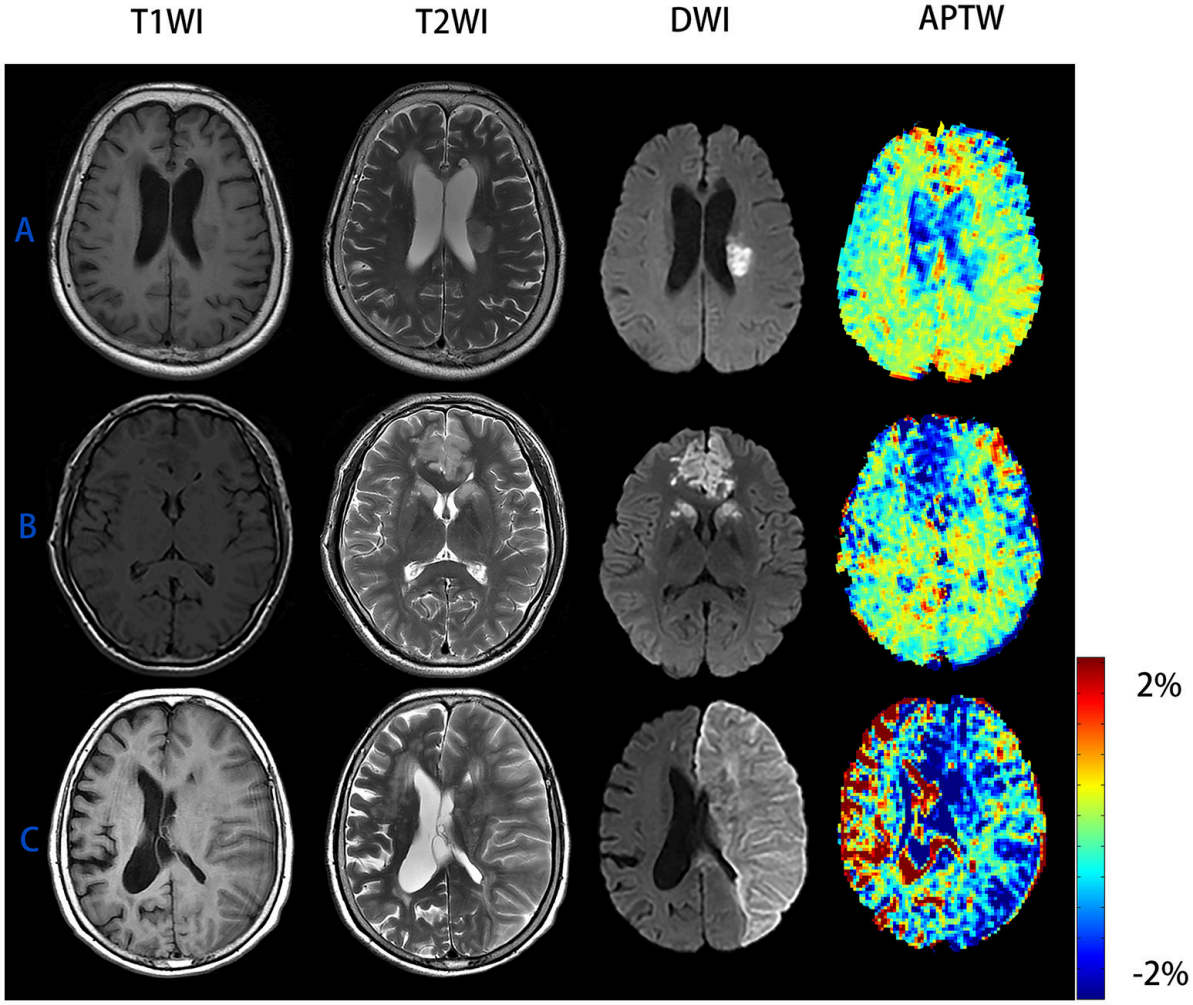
Conventional MR images and APTW images of patient with AIS of different NIHSS scores and mRS scores. (A) M/65 years, NIHSS score was 3 and 90-day mRS score was 0, ΔAPTW = −0.37%. (B) F/69 years, NIHSS score was 5 and 90-day mRS score was 2, ΔAPTW = 0.82%. (C) M/81 years, NIHSS score was 22 and 90-day mRS score was 6, ΔAPTW = 1.93%. The criterion for patient with good prognosis (mRS score <2) is ΔAPTW > −0.783.
